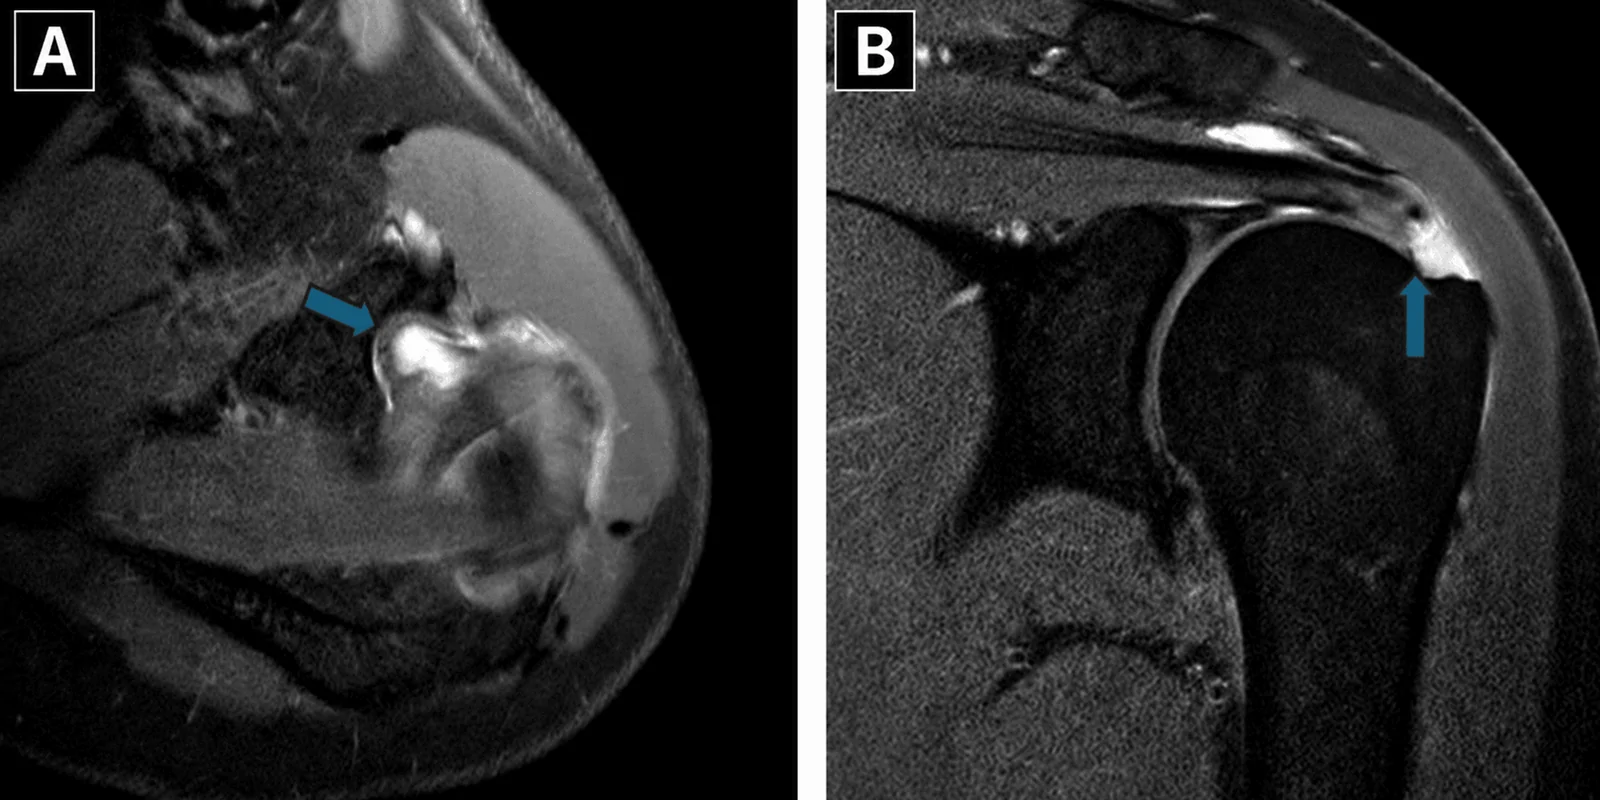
Full-thickness U-shaped supraspinatus tendon tear on MRI A: axial proton density fat-saturated MRI image demonstrates a full-thickness tear involving the distal supraspinatus tendon, with fluid signal extending through the tendon defect; B: coronal proton density fat-saturated MRI image shows transmural discontinuity of the supraspinatus tendon fibers at the footprint, with fluid signal extending from the articular surface to the bursal surface and associated subacromial-subdeltoid bursal fluid. The arrows indicate the site of tendon disruption.
